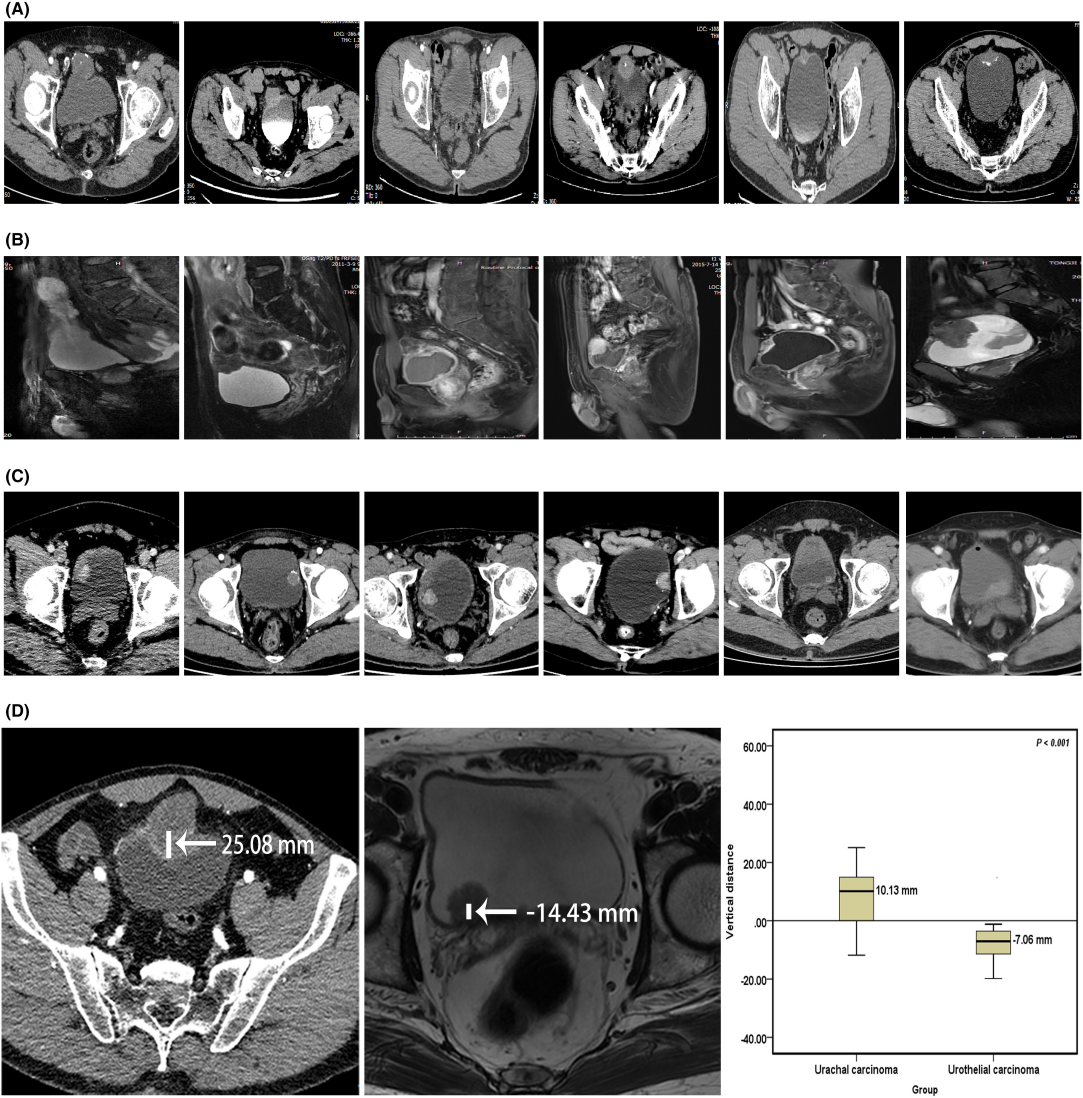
Comparison of imaging features between urachal carcinoma and urothelial carcinoma. (A) CT of urachal carcinoma. (B) MRI of urachal carcinoma. (C) CT of urothelial carcinoma. (D) Differences in tumor growth locations. CT, computed tomography; MRI, magnetic resonance imaging.
